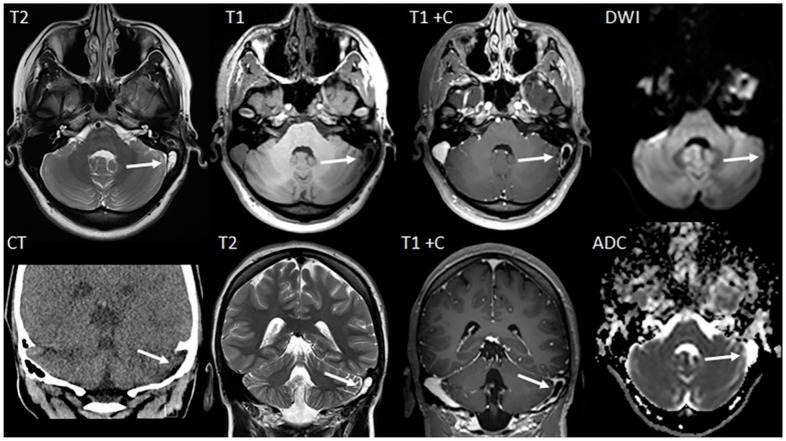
Axial MR images (T2-weighted, T1-weighted, T1-weighted with contrast, and DWI images) and matching coronal CT and MRI images (T2-weighted, T1-weighted with contrast, and axial ADC map) show a CSF-isointense, T2-hyperintense, T1-hypointense lesion in the left transverse/sigmoid sinus region (arrows). On the contrast enhanced T1-weighted sequence the lesion does not enhance. On DWI the lesion is DWI-hypointense with facilitated diffusion characteristics on the matching ADC map. The CT scan identifies this lesion as hypodense, while the MRI sequences confirm the diagnosis of a giant arachnoid granulation while excluding an endoluminal/dural sinus thrombus.
